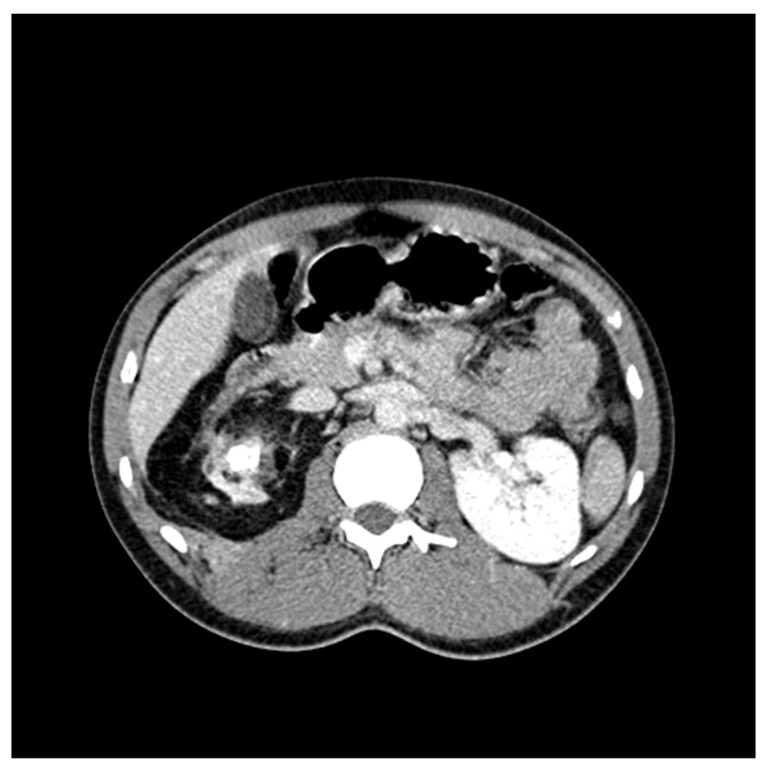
CT Scan showing perinephric edema mimicking pyelonephritis.
